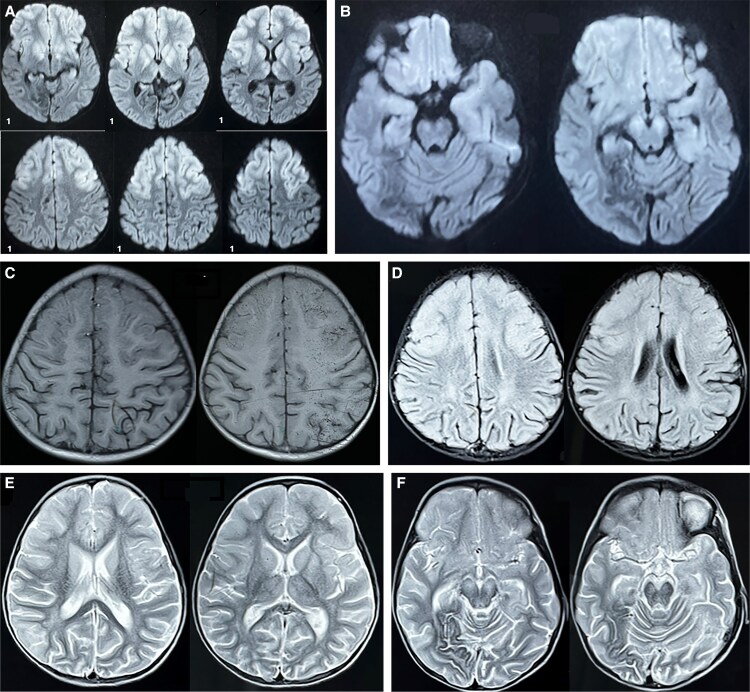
(*A, B*) Diffusion-weighted images show restriction in bilateral frontal cortex insula with bilateral basal ganglia, thalamus, and midbrain. (*C*) T1 image indicates hyperintensities in the bilateral frontal cortex. (*D–F*) T2/FLAIR images reveal white matter hyperintensities in bilateral frontal lobes with parietal and occipital lobes. Hyperintensities are also noted in bilateral basal ganglia, thalamus, and midbrain.
